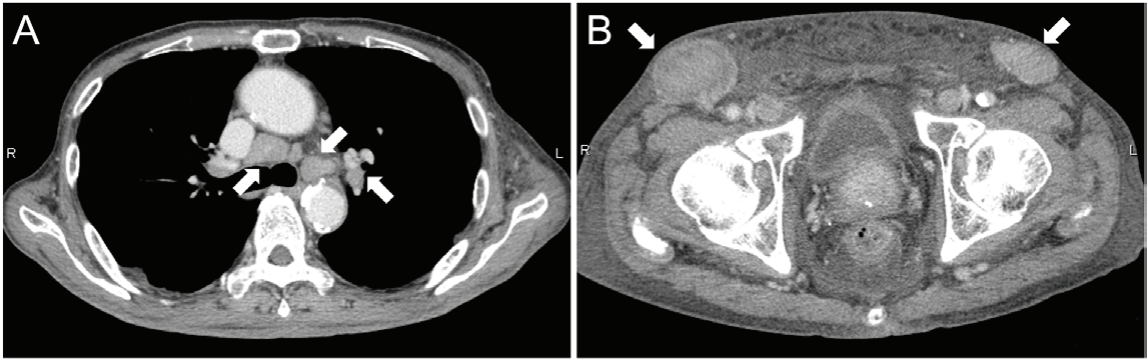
Computed tomography images. Multiple lymphadenopathies are observed in the hilar and mediastinal lymph nodes (A) and inguinal lymph nodes (B).
